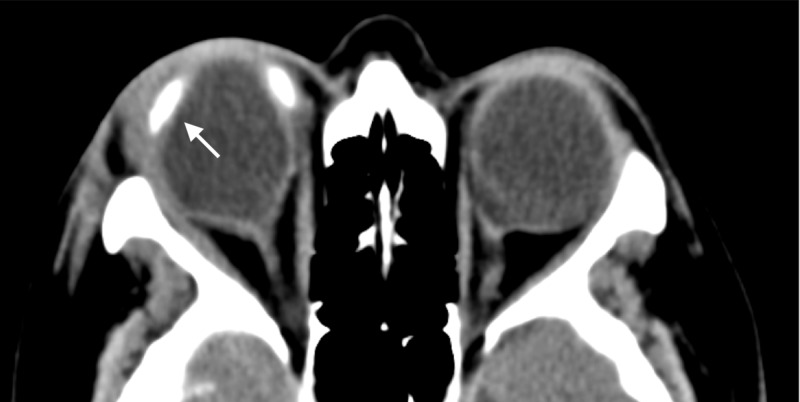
An axial CT image of the orbits. The radiopaque scleral buckle (white arrow) can clearly be seen encircling the right globe. There is no radiological evidence of any intra-orbital pathology; specifically no abscess or mass lesion. The corresponding ethmoid sinus is noted to be air-filled, without opacification.
